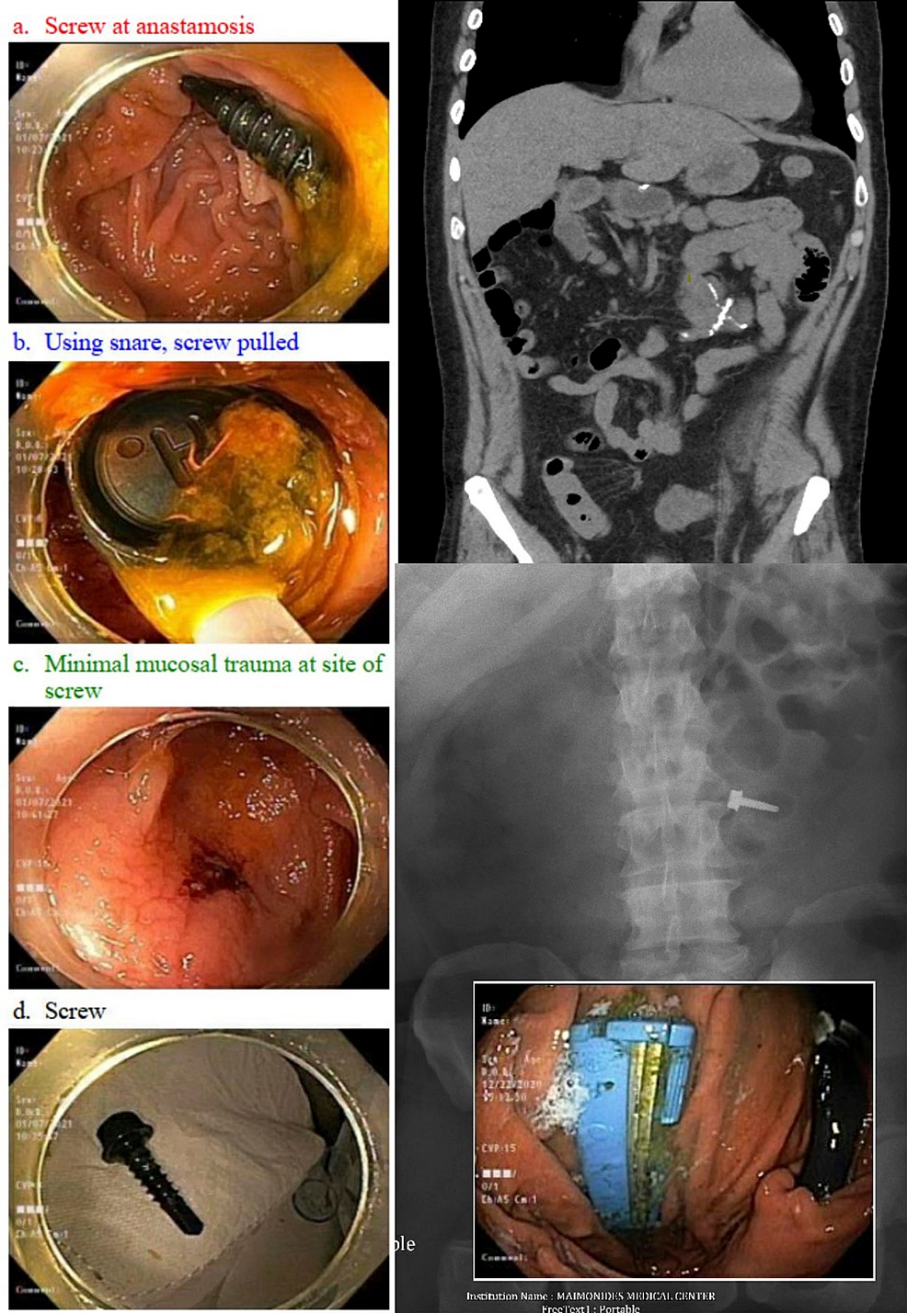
(Left panels) Colonoscopy and removal of foreign body, (top right) CT showing the previous anastomosis, (middle right) KUB showing the screw, (middle left) razor in fundus on EGD. KUB: radiograph of the kidneys, ureters, and bladder, EGD: esophagogastroduodenoscopy.
